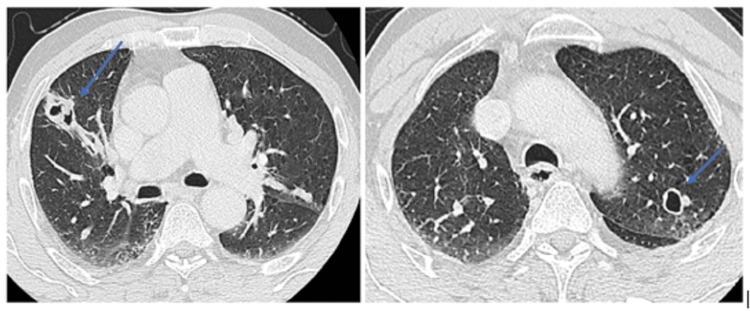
Axial thoracic CT scan showing bilateral pulmonary hydatid cysts with rupture and bronchial fistulization of the right hydatid cyst CT: computed tomography
